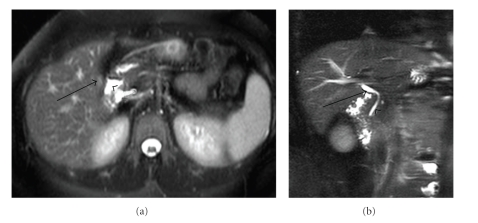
(a) Axial T2-weighted half-fourier acquisition single-shot turbo spin echo (HASTE) MRI image demonstrating a faint area of high signal in the area of the gallbladder fossa (arrow) adjacent to the second part of the duodenum (arrowhead). The radiological appearances are suggestive of a small contracted gallbladder. Although with the absence of a recognisable cystic duct, a surface haemangioma is a possible differential diagnosis. (b) T2 weighted coronal MRI image with fat suppression demonstrating a normal calibre extrahepatic biliary ducts (arrow). Note the absence of any cystic duct. Normal appearance of the pancreatic duct (arrowhead).
